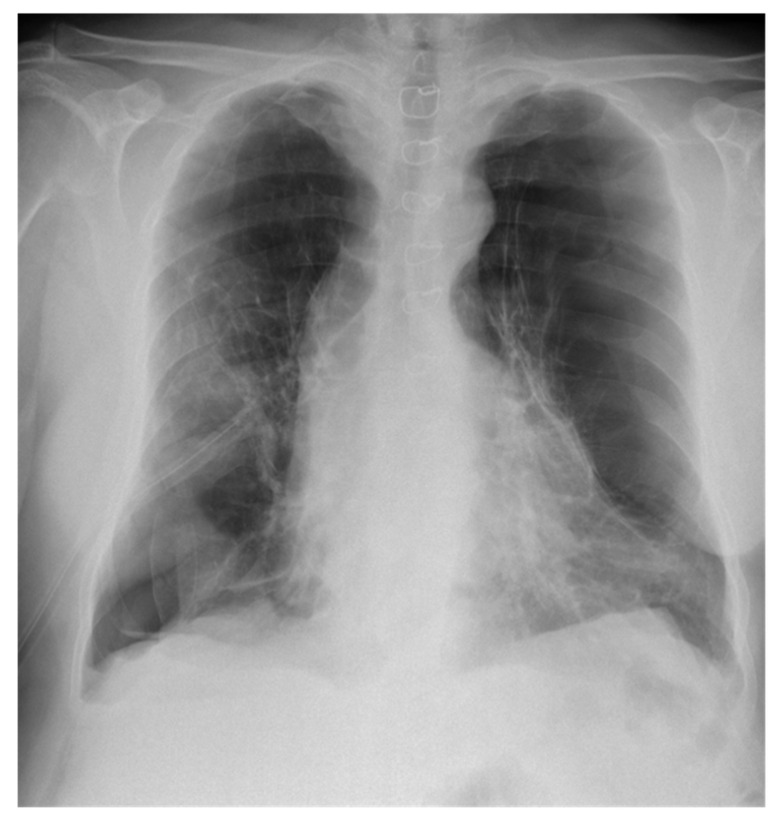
Posteroanterior chest X-ray showing limited expansion of the right lung day 4 after placement of the chest tube.
